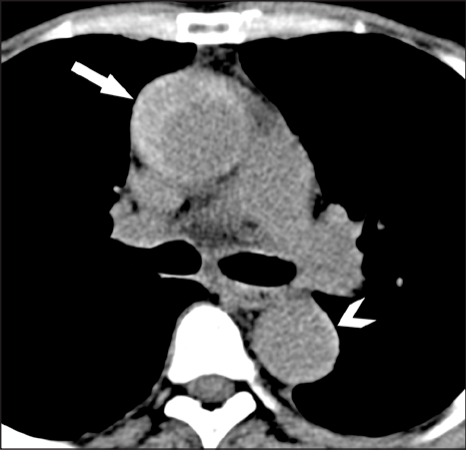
Unenhanced CT of the aorta in a 58-year-old woman with acute chest pain. A spontaneous dense semicircular structure can be seen in the ascending aorta, representing an acute intramural hematoma with wall thrombus (arrows in A-B). A small extension of this intramural hematoma can also be seen in the descending aorta (arrowhead in A).
